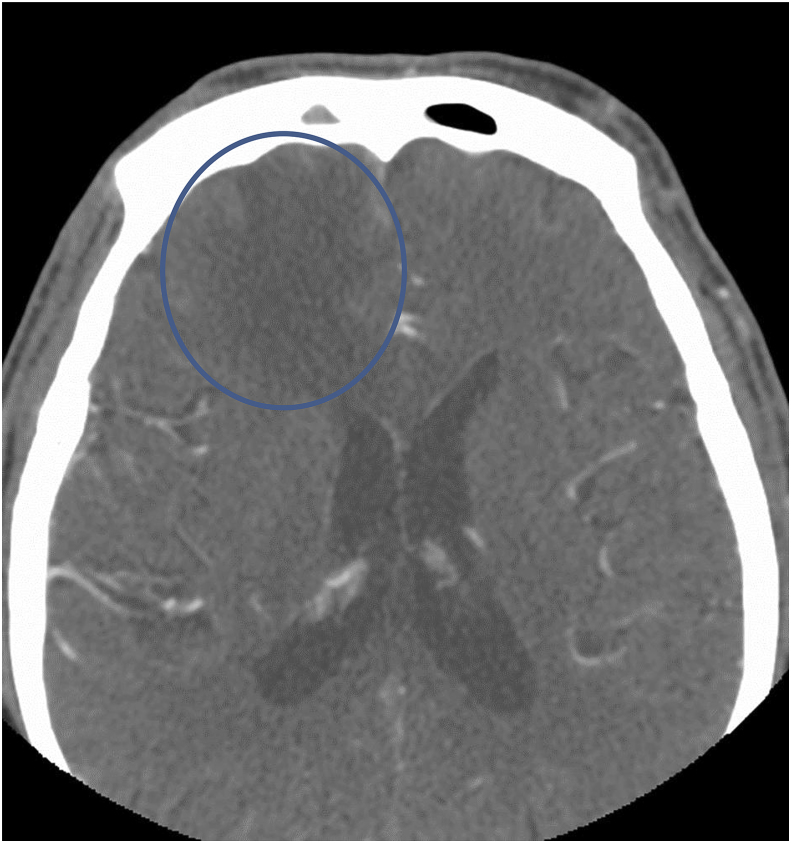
Axial CT scan of the brain with IV contrast showing vasogenic edema within the right frontal corona radiata and centrum semiovale. Mild mass effect on the frontal horns of both lateral ventricles.
